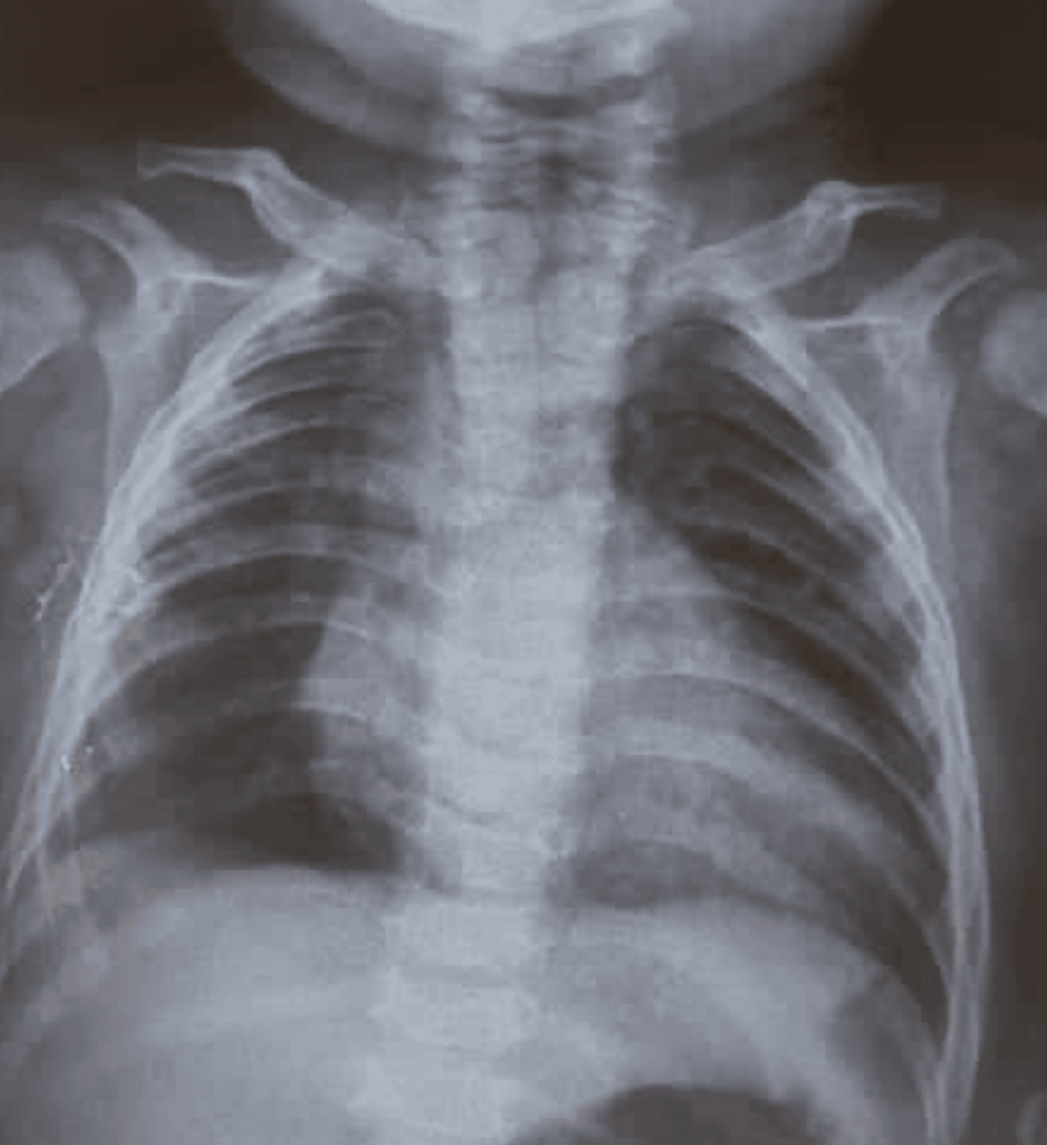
Chest radiography showing a boot-shaped cardiac silhouette
